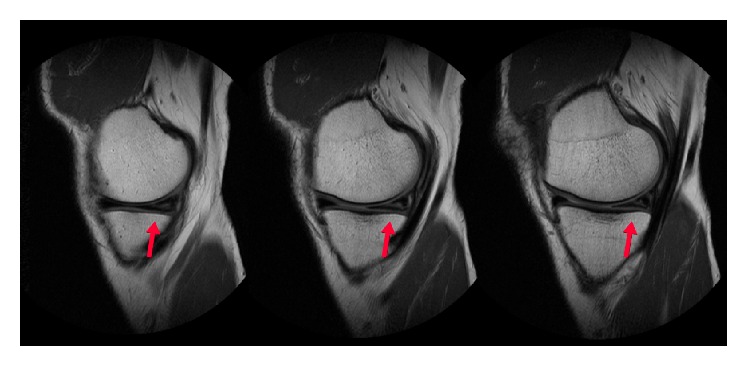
Sagittal Proton Density (PD) magnetic resonance imaging showing the chondral flap (arrow). It appears flap-like and is attached at its anterior aspect.
